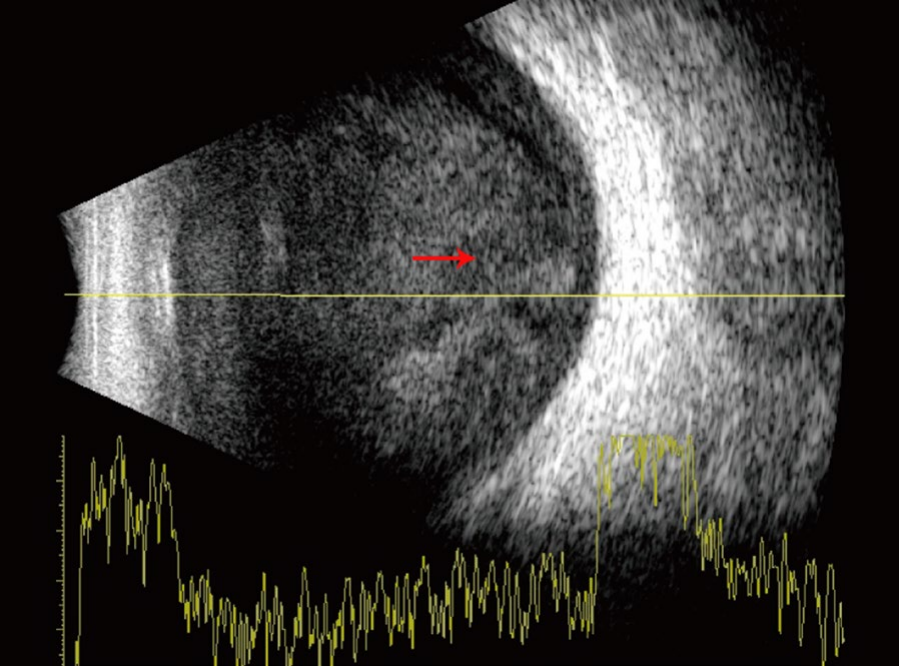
The disease aggravated progressively. Brightness scan ultrasound showed more dense vitreous debris (red arrow showing aggravated vitreous opacity) compared with Fig. 2b
